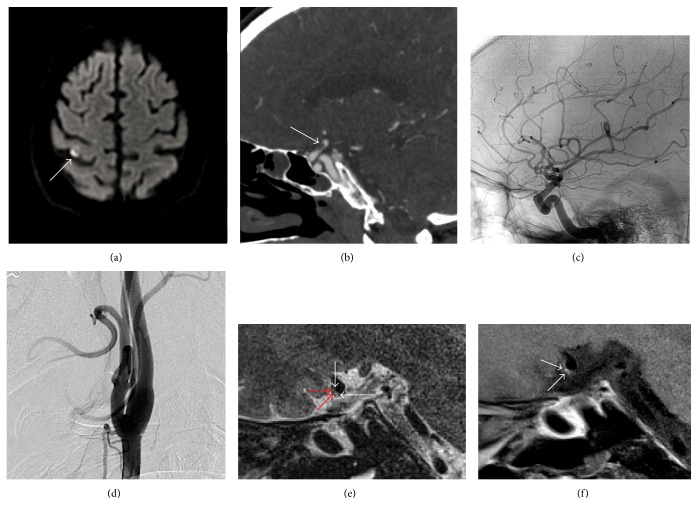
(a) Axial diffusion-weighted MRI showed punctate cortical areas of diffusion restriction (white arrow), consistent with an embolic source of ischemia. (b) Sagittal CT angiogram shows high-grade narrowing (white arrow) of the right supraclinoid ICA, presumed secondary to thrombus which was in close proximity to focal ICA calcification (not seen). (c) Lateral DSA performed two weeks after the prior CTA shows no significant stenosis or other vascular abnormality of the right anterior circulation. (d) Lateral DSA shows a patent right cervical internal carotid artery. (e) Sagittal T2-weighted HR-MRI of the supraclinoid ICA shows atherosclerotic plaque along the anterior wall of the supraclinoid ICA, with discontinuous juxtaluminal T2 hyperintense band (white arrows), and deeper T2 hypointensity representing the lipid necrotic core (red arrows). (f) Sagittal T1 postcontrast HR-MRI at the same level shows T1 hypointense plaque with focal enhancement along the juxtaluminal surface, which was not present on the precontrast T1 image, and a normal to eccentrically enlarged vessel lumen (white arrow).
